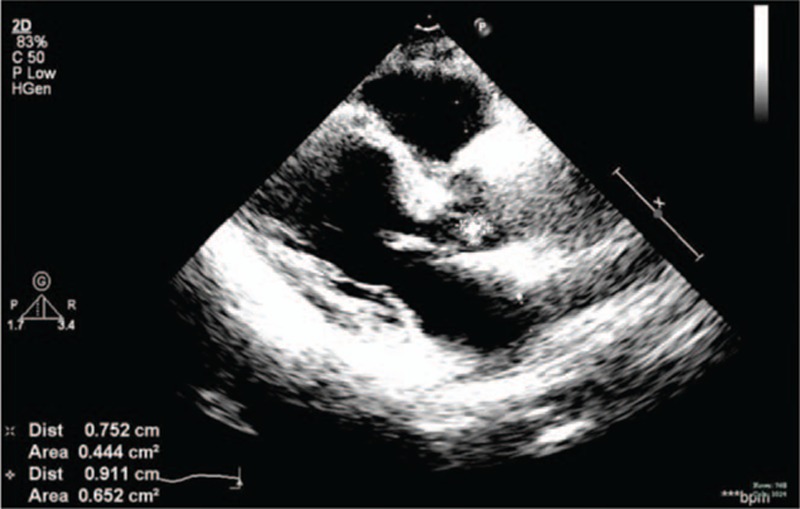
The echocardiogram presented a 0.91∗0.75 cm strong echo which was attached to the valvula coronaria sinistra valvae aortae.
